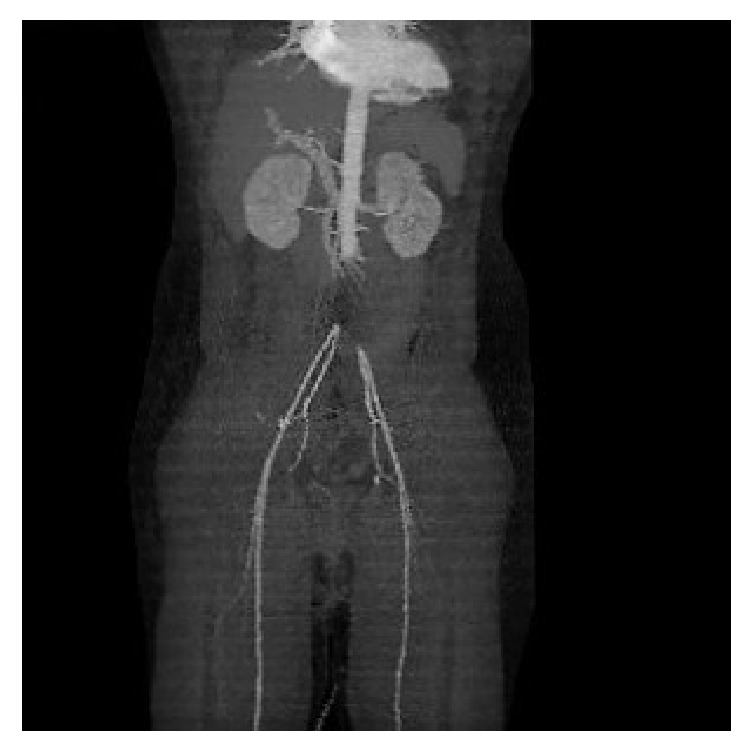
CT angiography depicted occlusion of infrarenal aorta and aortoiliac bifurcation.
